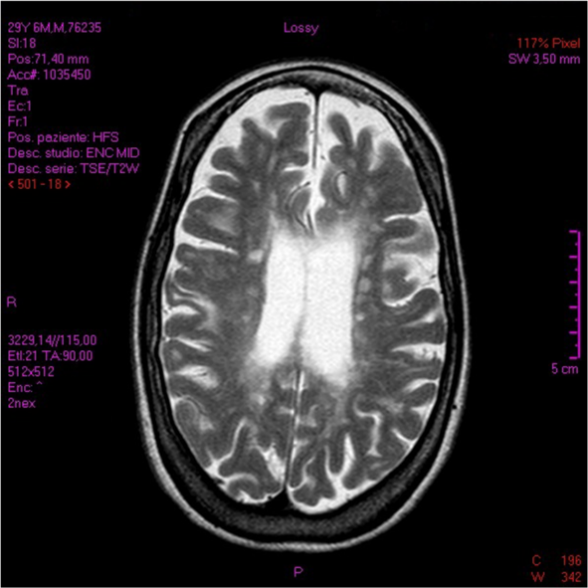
The brain MRI at baseline represented a moderate T2 lesion load
